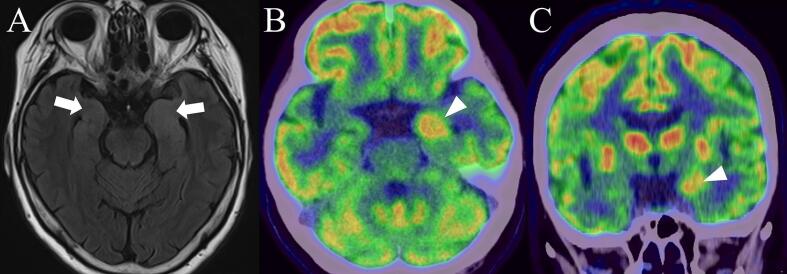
Brain magnetic resonance imaging and [^18^F]fluorodeoxyglucose ([^18^F]FDG) positron emission tomography (PET). (A) Fluid-attenuated inversion recovery imaging showed high intensity and enlargement of bilateral amygdalae with left dominance (arrows). (B and C) [^18^F]FDG PET revealed increased uptake in the left amygdala (arrowheads).
